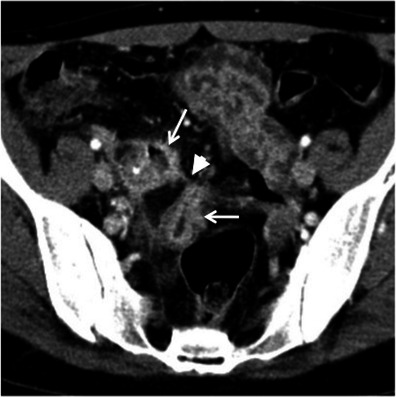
Stratified appearance in Crohn’s disease. Axial contrast-enhanced CT scan of the abdomen shows concentric wall thickening of small bowel loops with a stratified appearance indicating active disease (*arrows*). Also note a fistula (*arrowhead*) connecting the bowel loops, a common finding in Crohn’s disease
